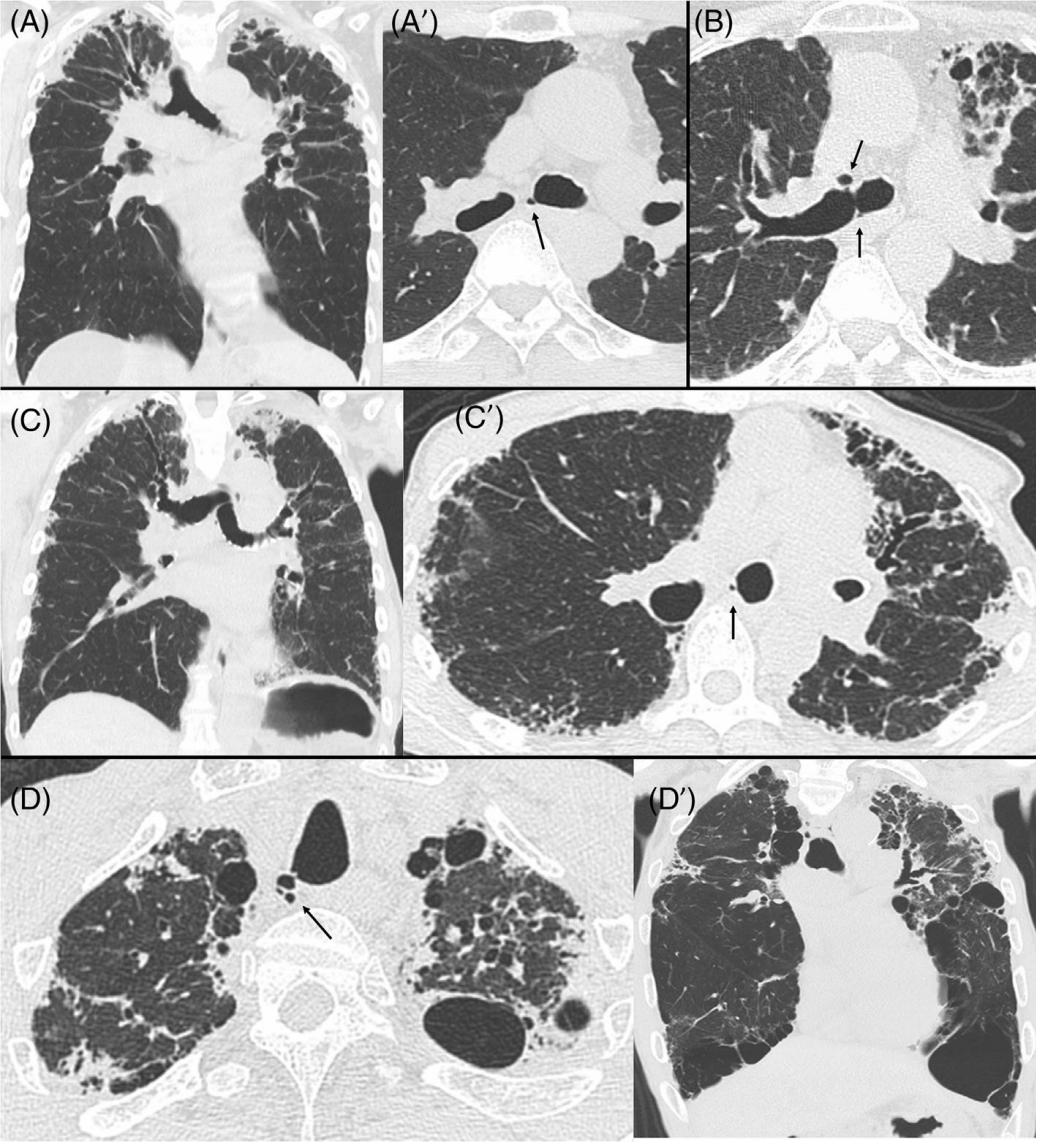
(A/A′) Coronal and axial HRCT images of the chest of a 66‐year‐old woman with PPFE show a small air collection communicating with the left main bronchus (arrow). (B) Chest HRCT image of a 60‐year‐old woman with PPFE shows para‐carinal air cysts in both the right (arrow) and left (arrow) bronchi. (C/C′) Coronal and axial HRCT images of the chest of a 66‐year‐old man show upper lobe‐dominant fibrosis with a para‐carinal air cyst (arrow). (D/D′) Coronal and axial HRCT images of the chest of a 75‐year‐old man with PPFE show a small air collection as a para‐tracheal air cyst (arrow) communicating with the trachea.
